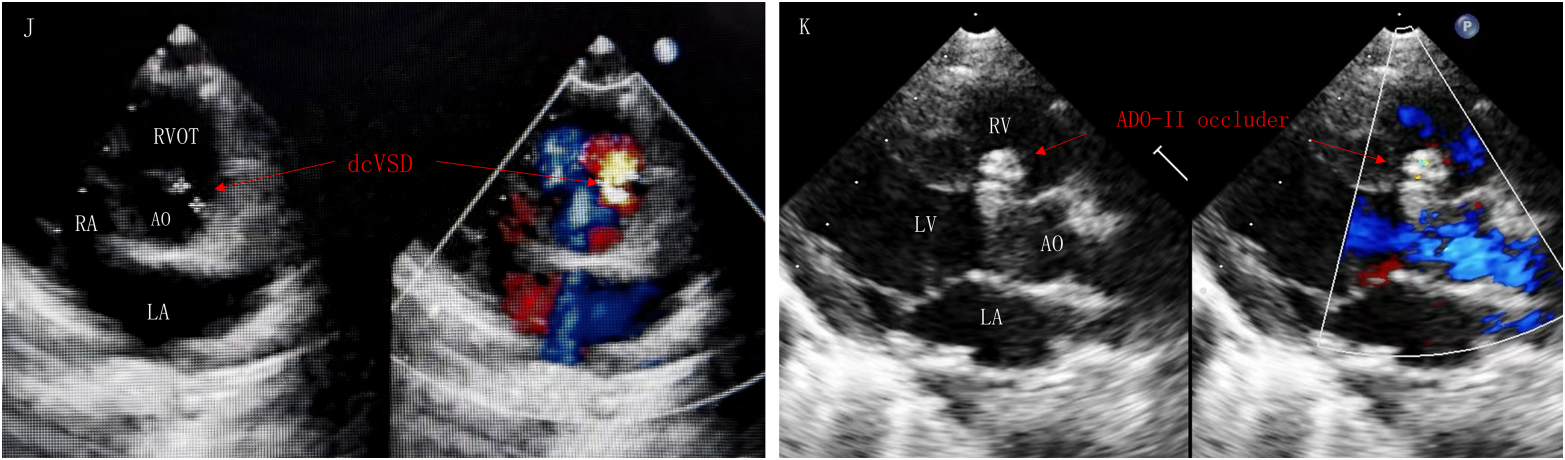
Parasternal short-axis view of the great vessels (J): postoperative TTE showed dcVSD. Left sternal border long-axis view of the left ventricle (K): dcVSD was successfully occluded by ADO-II occlude without AR and RS. AR, aortic regurgitation; AO, aorta; LA, left atrium; LV, left ventricle; RV, right ventricle; RS, residual shunt; RVOT, right ventricular outflow tract.
